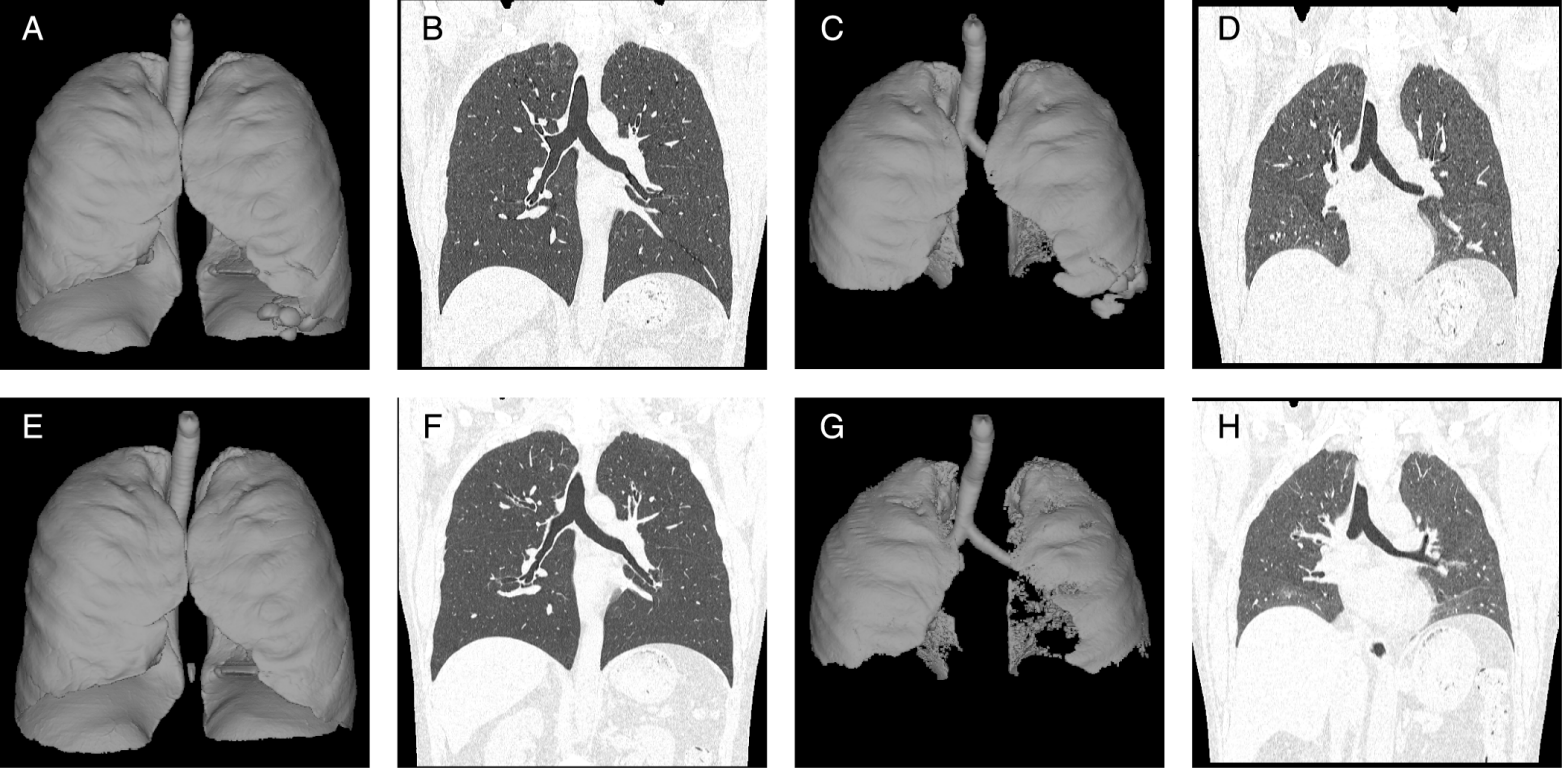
Lung capacity by Virtual Place in the inspiration and expiration. Case: A 44‐year‐old man; asthma disease duration: eight years; lung total volume on inspiration before bronchial thermoplasty (BT): 5873 mL. (A) Computed tomography (CT) coronal slices on inspiration before BT; (B) lung total volume on expiration before BT 2226 mL; (C) CT coronal slices on expiration before BT; (D) lung total volume on inspiration after BT 5660 mL; (E) CT coronal slices on inspiration after BT; (F) lung total volume on expiration after BT 1067 mL; (G) CT coronal slices on expiration after BT (H).
